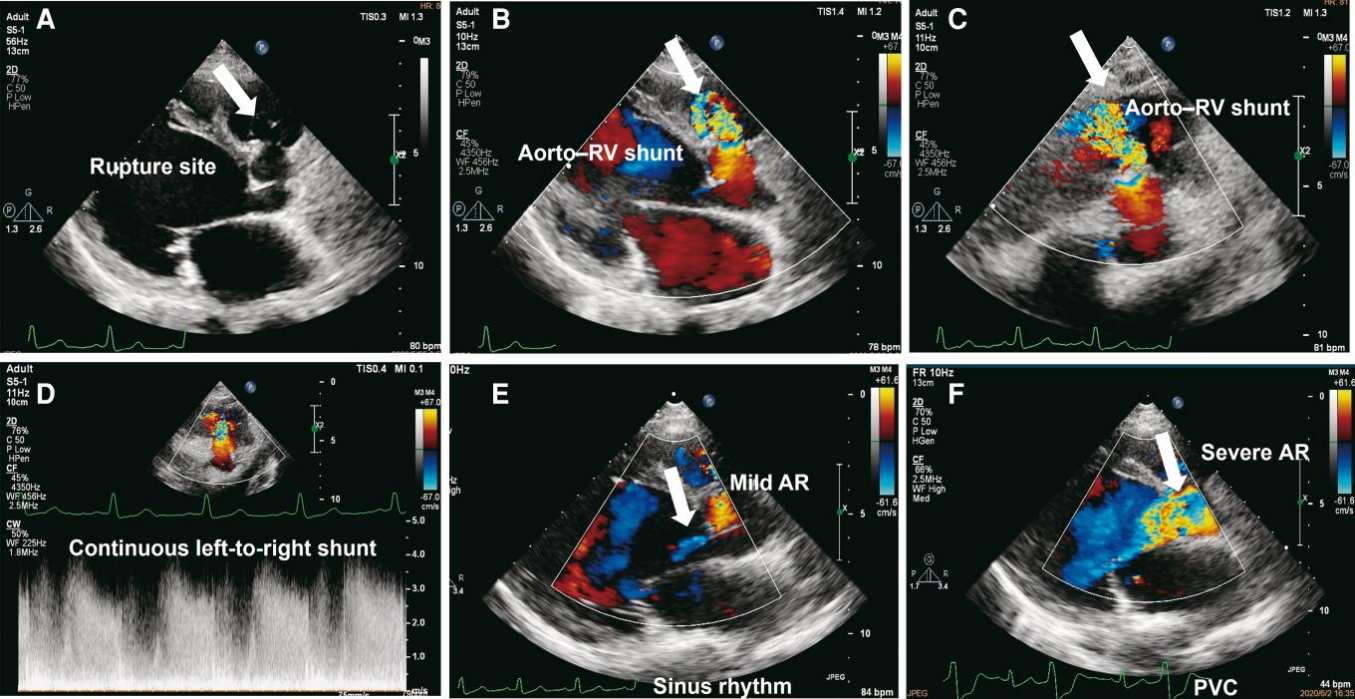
Transthoracic echocardiography (TTE) shows a ruptured right sinus of Valsalva aneurysm (RSVA) protruding into the right ventricle with continuous left-to-right shunting (*A–D*). In the same colour Doppler plane, aortic regurgitation is mild during sinus rhythm (*E*) but becomes severe during a premature ventricular contraction (*F*).
